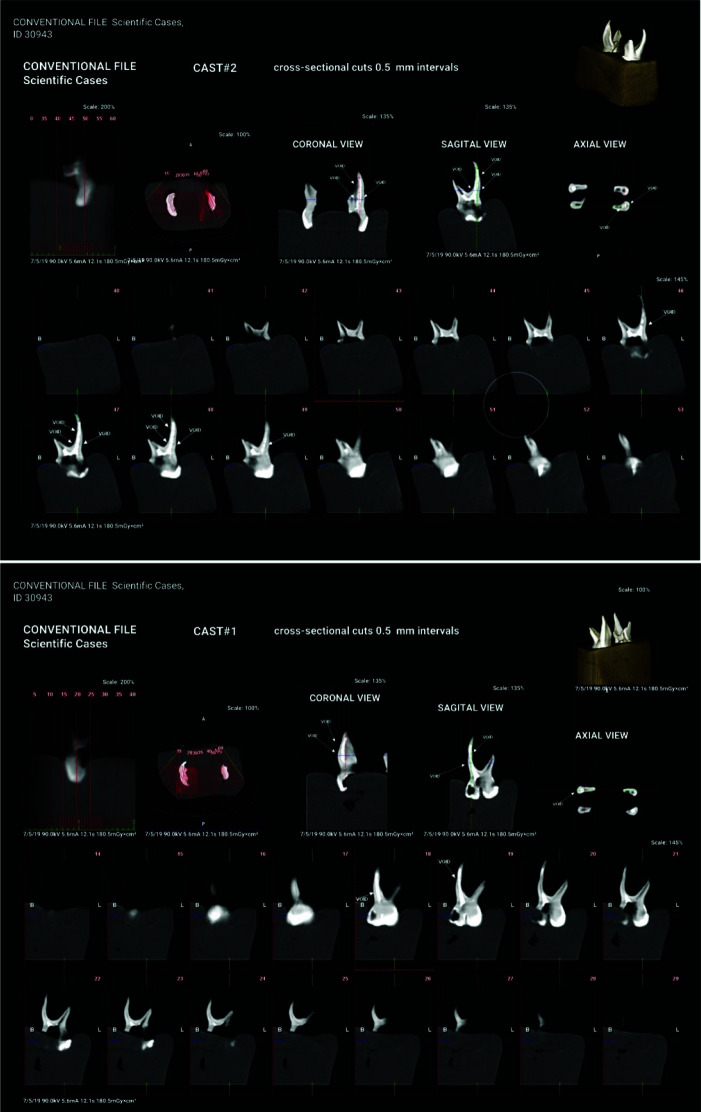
Cone beam computed tomography (CBCT) for assessment of root canal obturation quality
